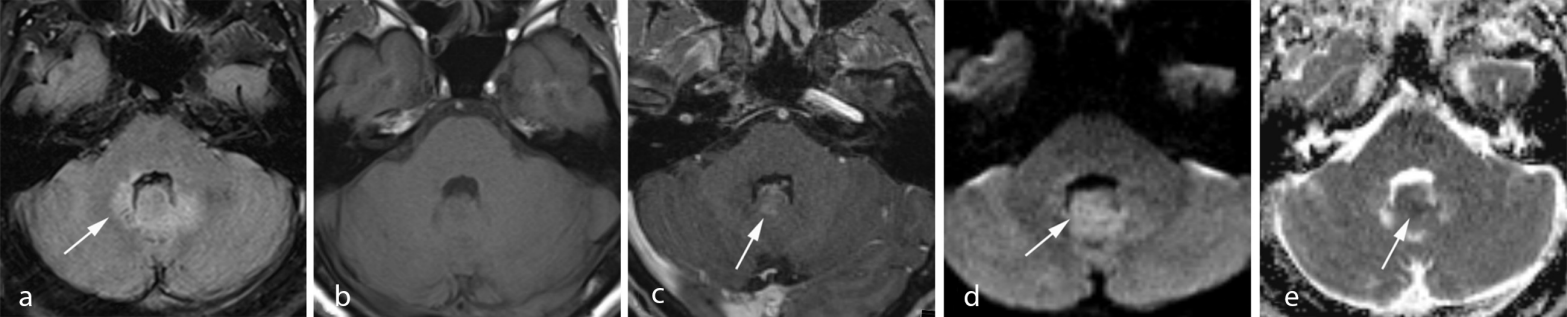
MR images at the time of diagnosis. *T*_2_ fluid-attenuated inversion recovery image on which the abnormal *T*_2_ hyperintense signal on the dorsolateral side of the fourth ventricle and hyperintense nodulus of the vermis can be appreciated (a, arrow). Being isointense to surrounding white matter on *T*_1_ weighted images (b) the area showed subtle, nodular-like contrast enhancement (arrows in *T*_1_ weighted postcontrast image c). Diffusion-weighted imaging showed subtle diffusion restriction (arrows in d (diffusion-weighted image) and e (apparent diffusion coefficient map)).
